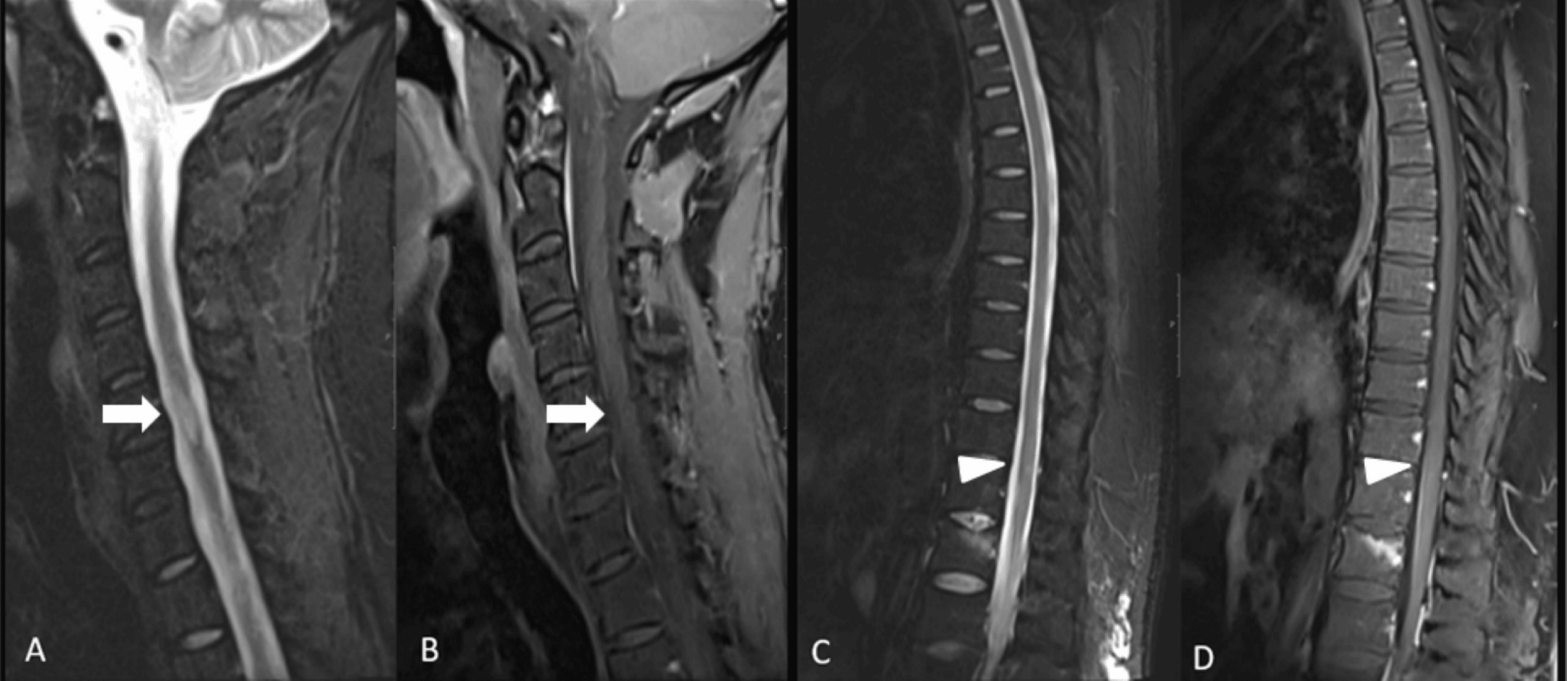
MRI of the cervical and thoracic spine. STIR images (A, C) and post-contrast T1-weighted images (B, D) reveal a non-enhancing demyelinating lesion in the peripheral spinal cord at the C5–C6 level (arrow in A; A-B). Additionally, subtle patchy lesions are observed throughout the thoracic spinal cord (arrowhead in C; C-D), without contrast enhancement or cord expansion. STIR: short tau inversion recovery.
